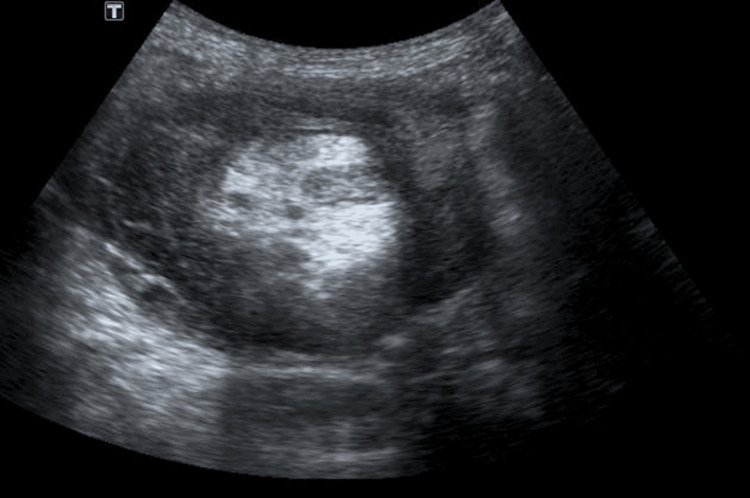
Ultrasonography of the abdomen.
